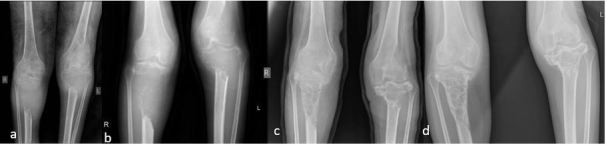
Knee radiographs showing (a) lytic lesions with soft tissue components in the bilateral proximal tibia and to a lesser extent the distal left femur with progressive bone formation in (b) after 1 cycle of chemotherapy (c) after 2 cycles of chemotherapy and (d) post-radiotherapy and 4 cycles of chemotherapy.
